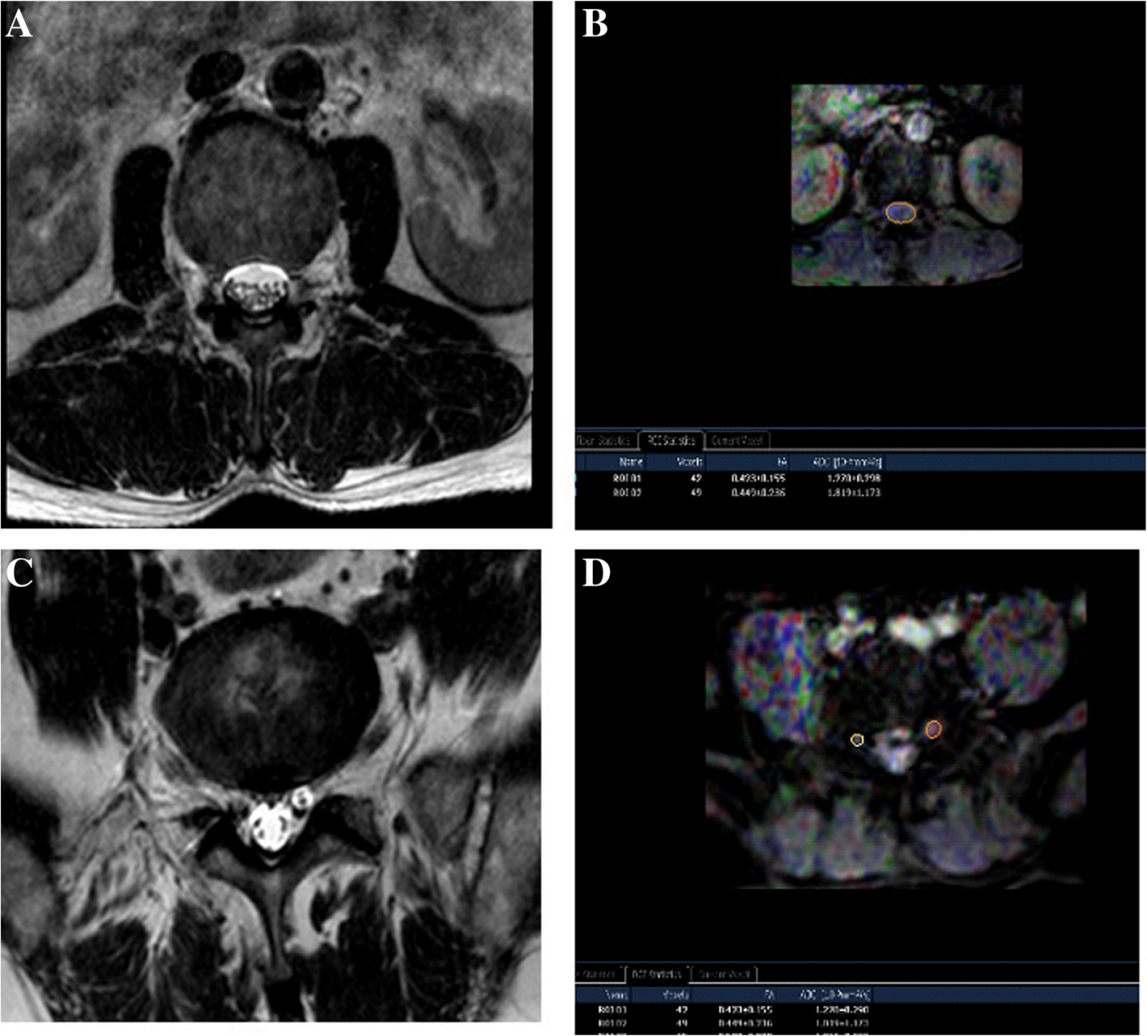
The cauda equina MRI T2W image (a) and its FA mapping of DTI (b). ROIs were placed on the cauda equina on the zones equally to the disc plane (superior 1/3, middle1/3, and inferior 1/3) on the FA mapping, and the FA values were calculated (b), the minimum value of three zones was taken as the cauda equina FA at that level. MRI T2W image of bilateral nerve roots (c) and FA mapping of DTI of bilateral nerve roots (d). ROIs were placed on the:“intraspinal,” “intraforaminal,” and “extraforaminal” zones of bilateral nerve roots on FA mapping and the FA values were calculated (d), the minimum value of three zones was taken as the nerve root FA value. MRI, magnetic resonance imaging; FA, fractional anisotropy; DTI, diffusion tensor imaging
